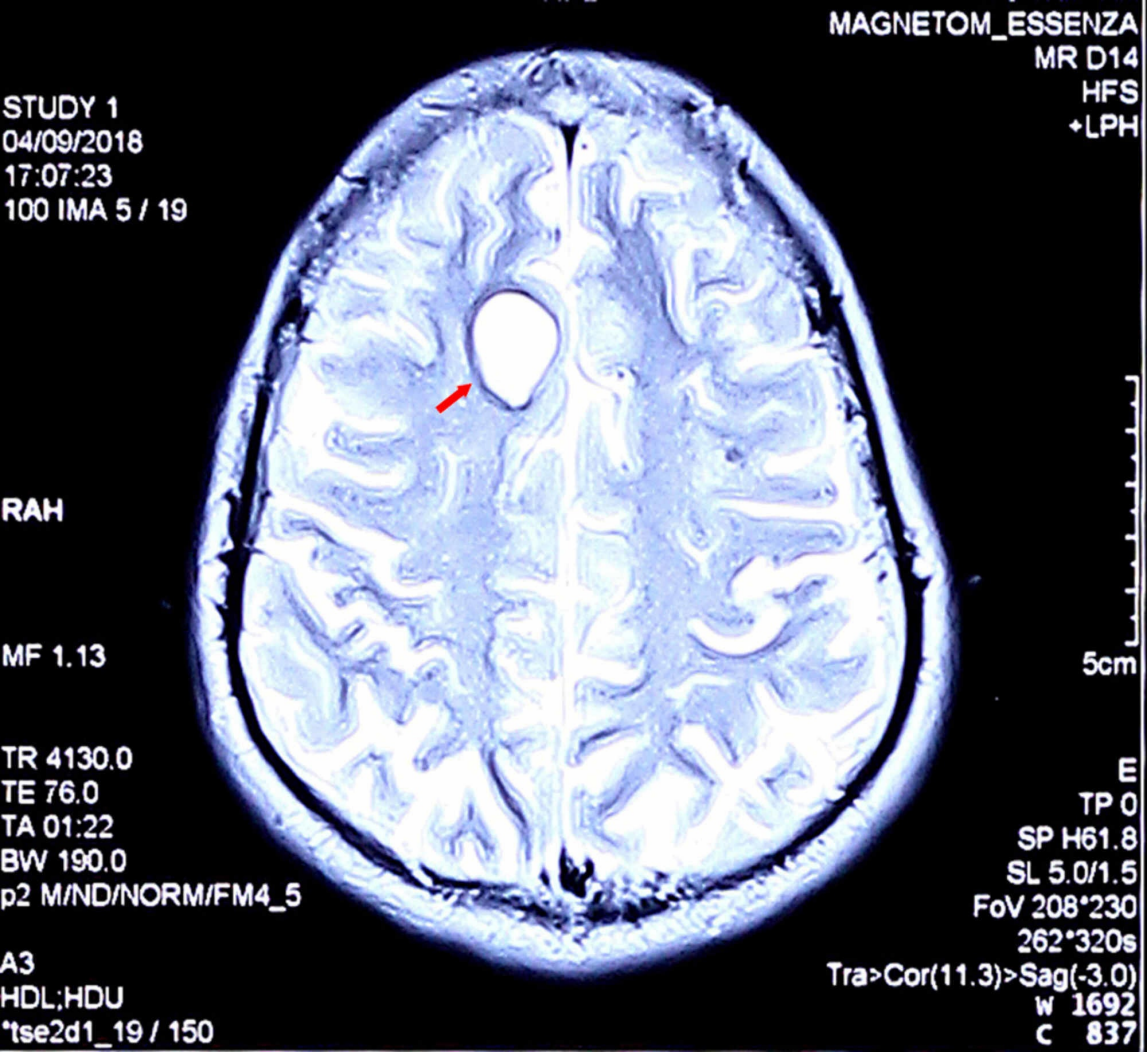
MRI axial T2 weighted image of case 1 The axial T2 weighted image showed a hyperintense, well-defined border cystic lesions in the right frontal lobe with a diameter of 11 mm MRI: magnetic resonance imaging
